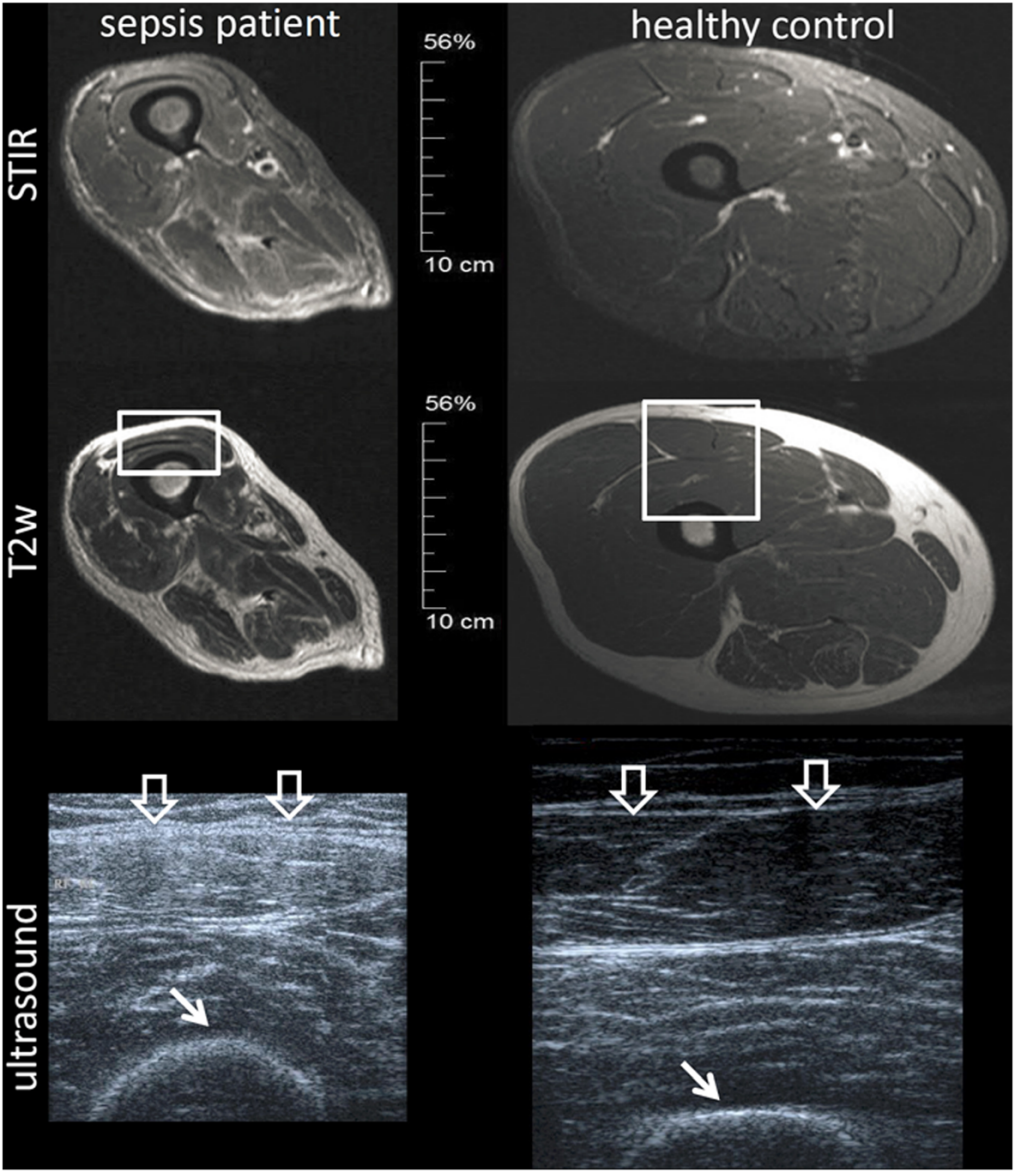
Muscle magnetic resonance imaging (1.5 Tesla) of a sepsis patient and a healthy control. Left: sepsis patient at day 14. Right: healthy control. Short TI inversion recovery (STIR) is a magnetic resonance imaging (MRI) sequence to suppress the signal from fat, so that especially edema can be seen in the muscle. The images demonstrate that at day 14 after onset of severe sepsis the structural changes in the muscle (atrophic and fibrous changes) dominate over edematous changes. Ultrasonic sections of the same subjects through the rectus femoris muscle (large arrows) are shown at the bottom. The corresponding areas in MRI are marked as rectangles. The difference in echogenicity of the muscle can clearly be seen. Moreover, the bone signal in the patient begins to blur (small arrows).
